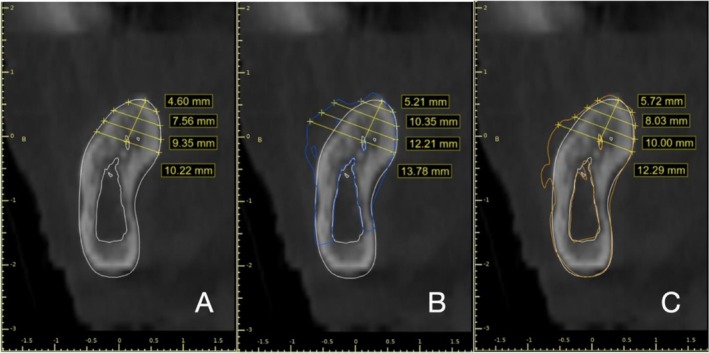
Example of a central linear measurement of the periosteal suture (PS) fixation technique at 1, 3, 5, and 7 mm from the crest. (A) Baseline CBCT. (B) Pre‐closure bone surface aligned to baseline CBCT. (C) Post‐closure bone surface aligned to baseline CBCT.
